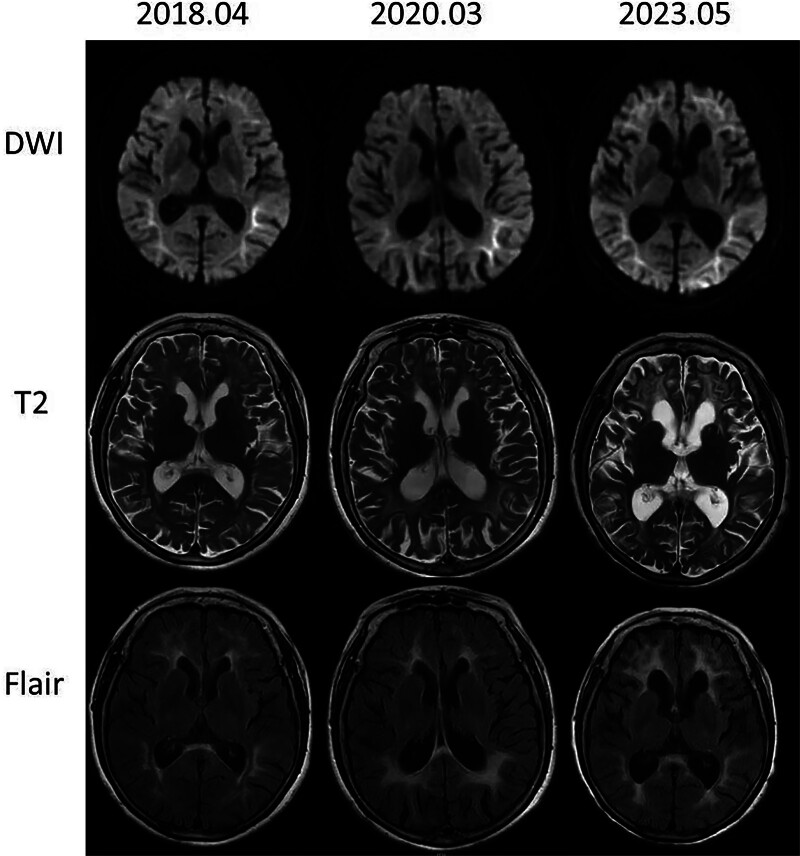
Diffusion-weighted imaging (DWI) showing subcortical hyperintense signals along the junction area of the cortex and white matter. T2 and fluid-attenuated inversion recovery (FLAIR) images exhibiting progressive diffuse confluent white matter changes.
